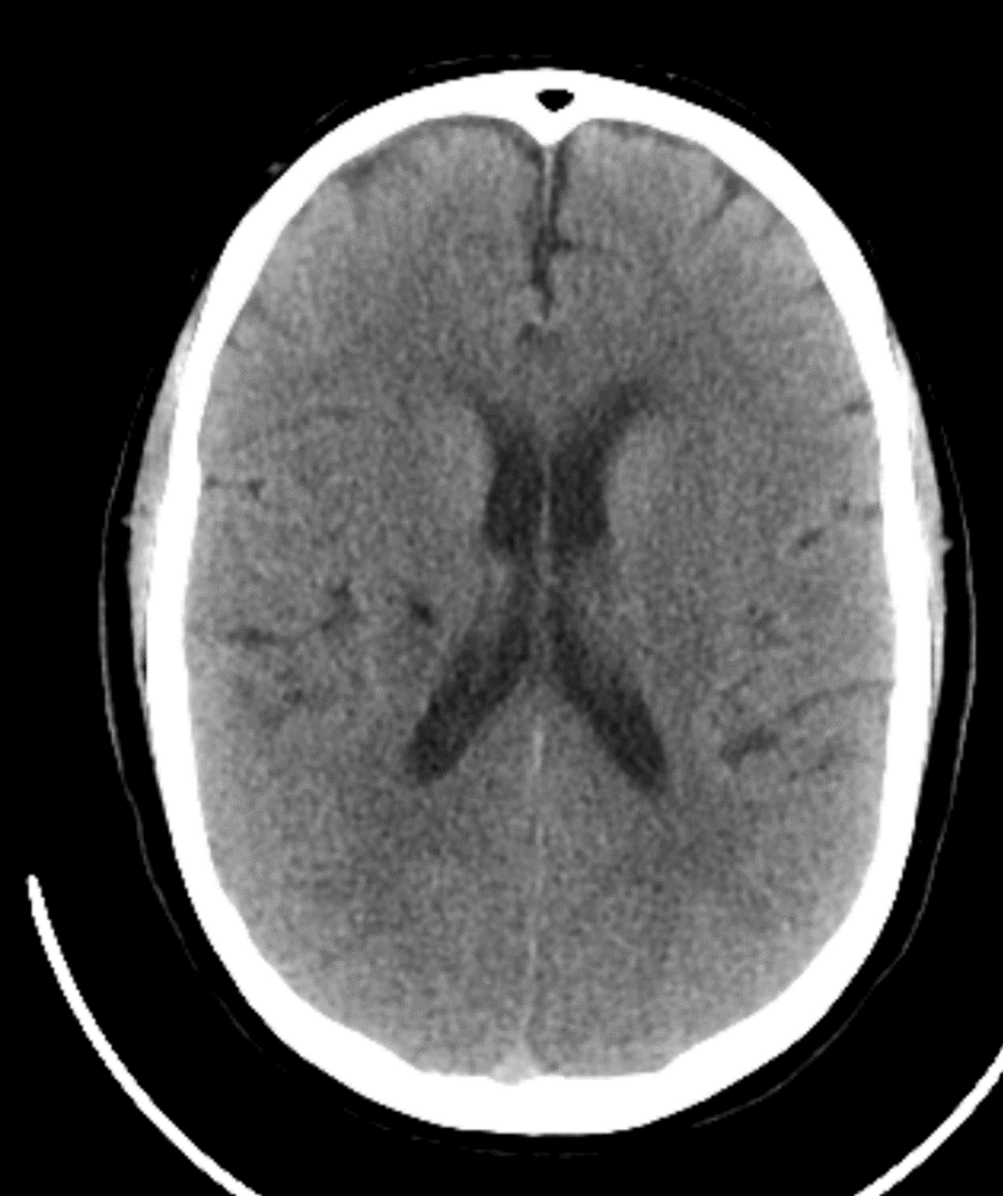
24-hour post-TNK CT head imaging showing no evidence of hemorrhagic transformation CT: computed tomography; TNK: tenecteplase
